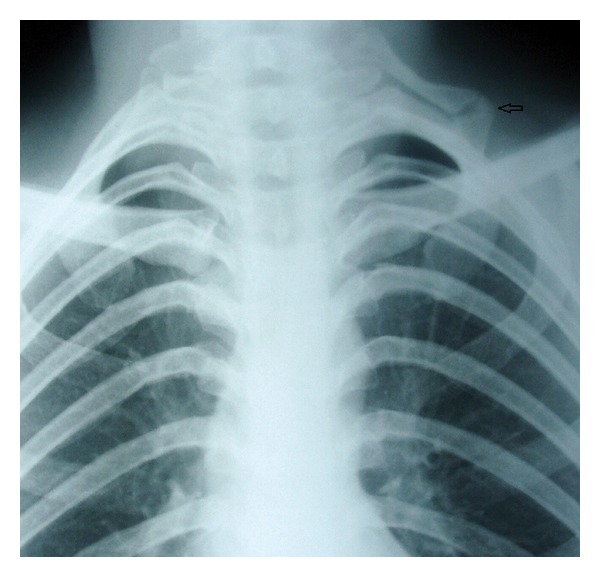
X-ray of chest and cervical spine (PA view) showing left cervical rib with fracture (arrow).
